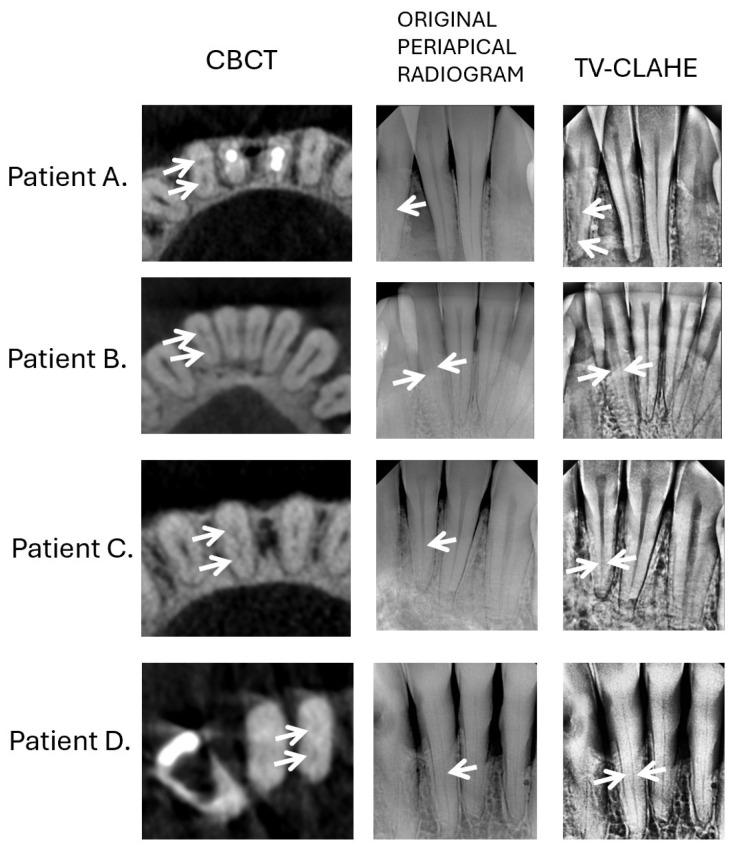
Comparison of root canal visualization in four patients (Patient A–D). Left column: axial CBCT images; middle column: original periapical radiographs; right column: images enhanced using the proposed TV-CLAHE method. All examples show mandibular lower incisors in the axial (CBCT) and coronal (periapical) planes. Arrows indicate root canals, with enhanced visibility in the processed images.
